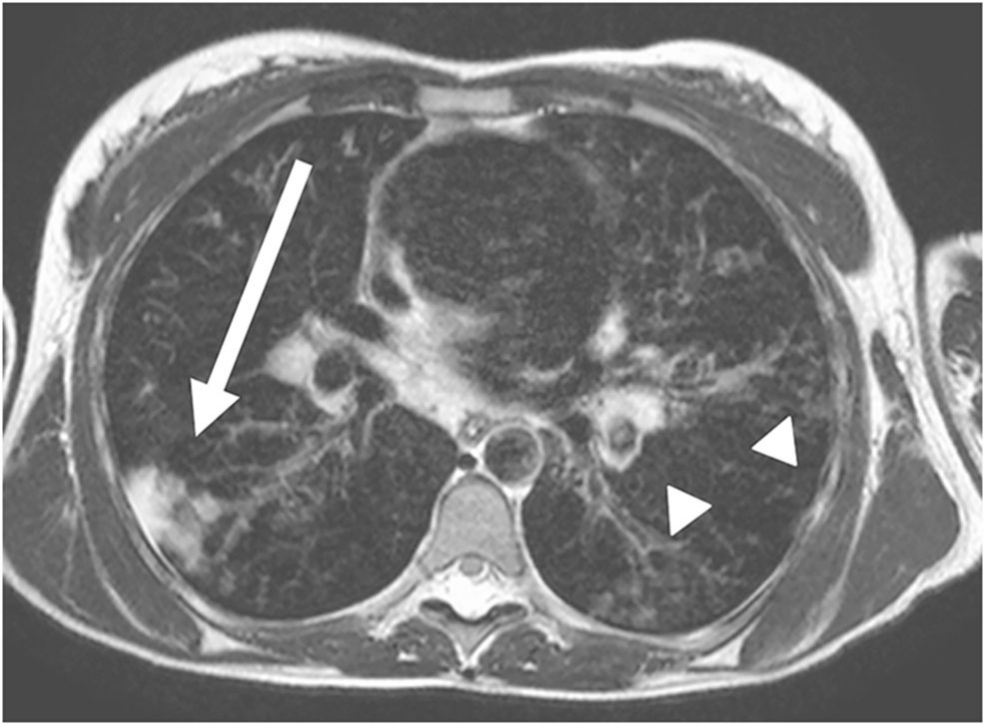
Bronchopneumonia on lung MR in a 10-year-old girl (axial T2-weighted turbo spin echo, parameters in Table 2). There is alveolar exudation and infiltration in bronchopneumonia in the right lower lobe (*arrow*) and, to a lesser extent, in the left lower lobe (*arrowheads*)
